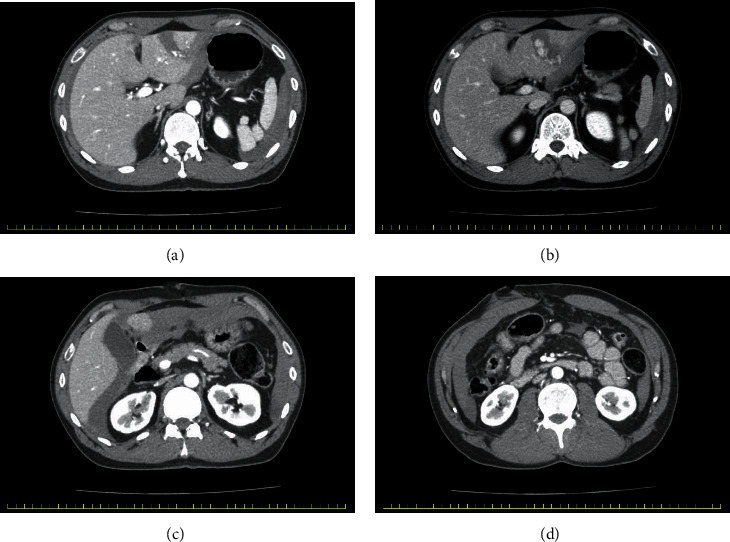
Contrast-enhanced computed tomography of the abdomen, taken before the initial operation, shows a laceration of the left lateral segment of the liver and extravasations (a, b), hematoma in the Morrison's pouch (c), and the penetration area (d).
